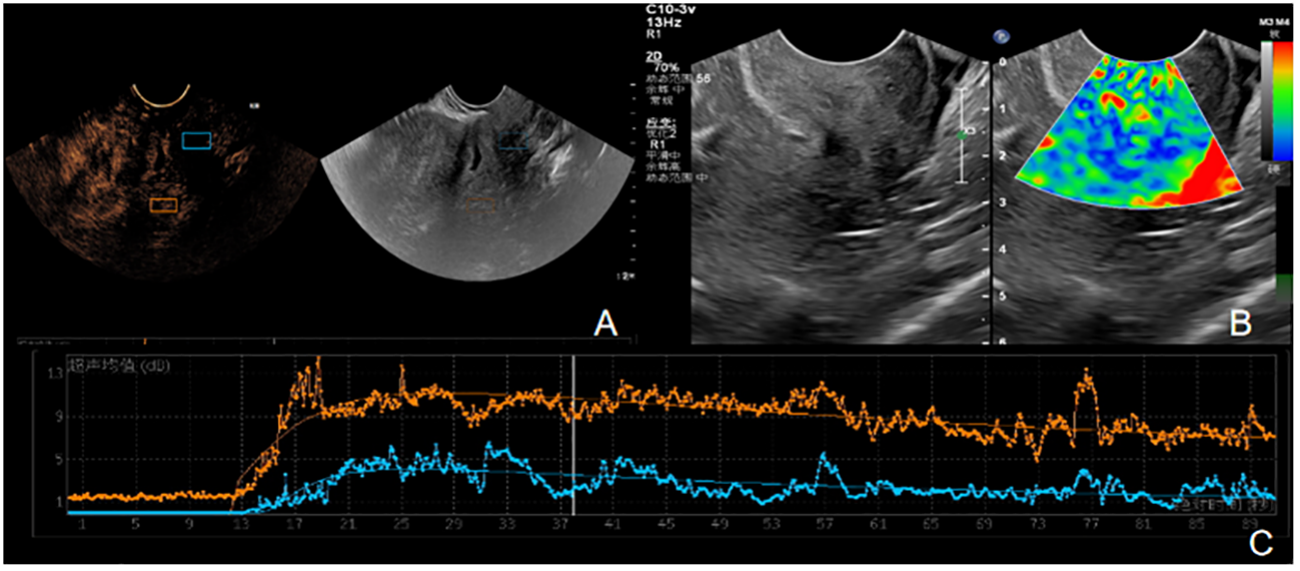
(A-C) is the contrast ultrasound and elastography of SD patients. (A) There was no obvious enhancement in the lesion area. (B) The elasticity of the lesion was soft, the center was blue and green, and blue was the majority. The elasticity score was 2, and the SR value was about 1.6. (C) TIC curve drawn after angiographic imaging of the lesion area. Blue represents TIC curve of the lesion area, and orange represents TIC curve of normal tissue at the same level of the lesion. The lesion PI was 9dB, TTP was 28S, and AUC was 521 DB.s.
